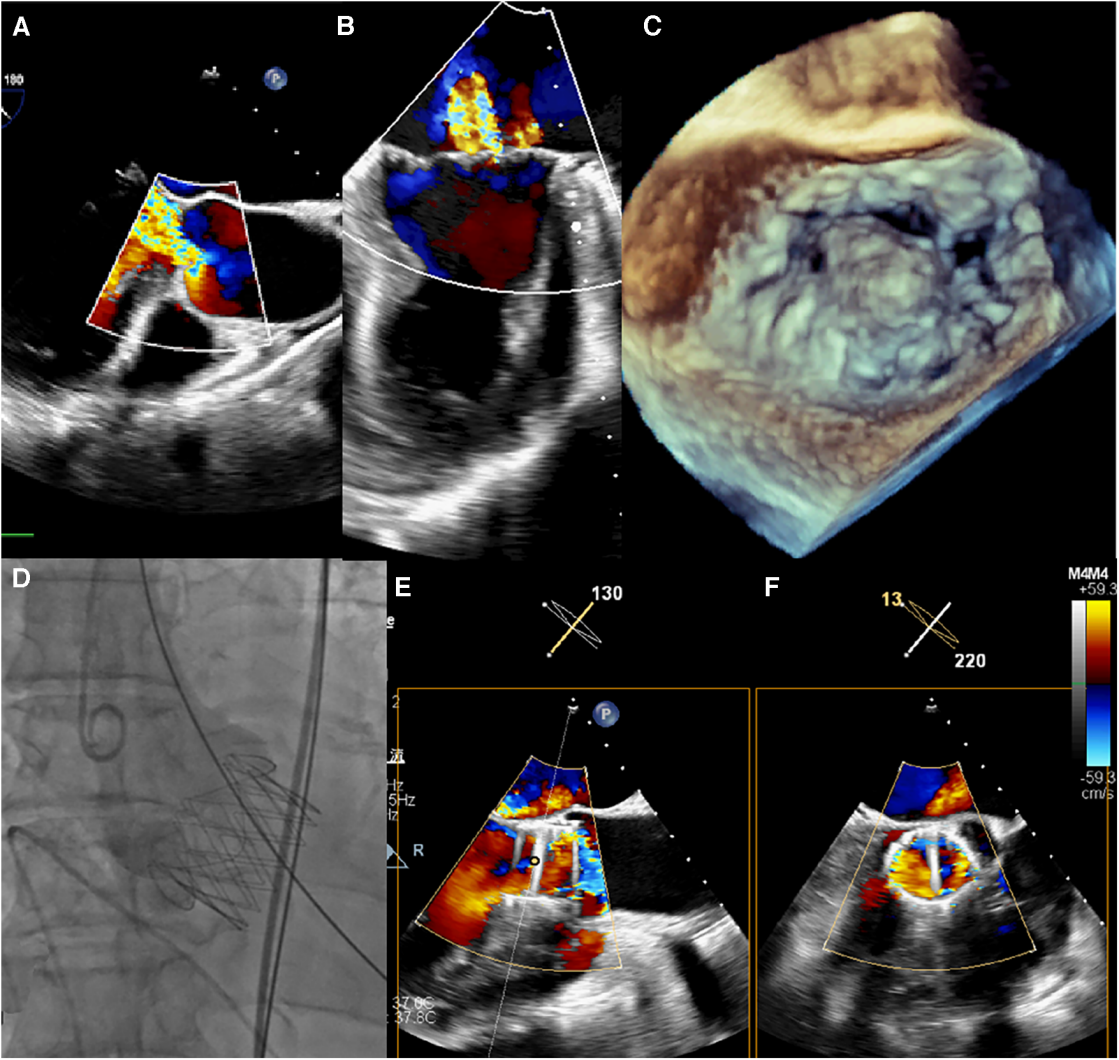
(A–C) intraoperative transoesophageal echocardiography confirmed severe aortic and MR. (D) Transapical TAVR was performed under fluoroscopic guidance using a 29 mm J-Valve (JieCheng Medical) as previously described, and aortic root angiography revealed minimal regurgitation. (E,F) After TAVR, the grade of MR remained severe.
